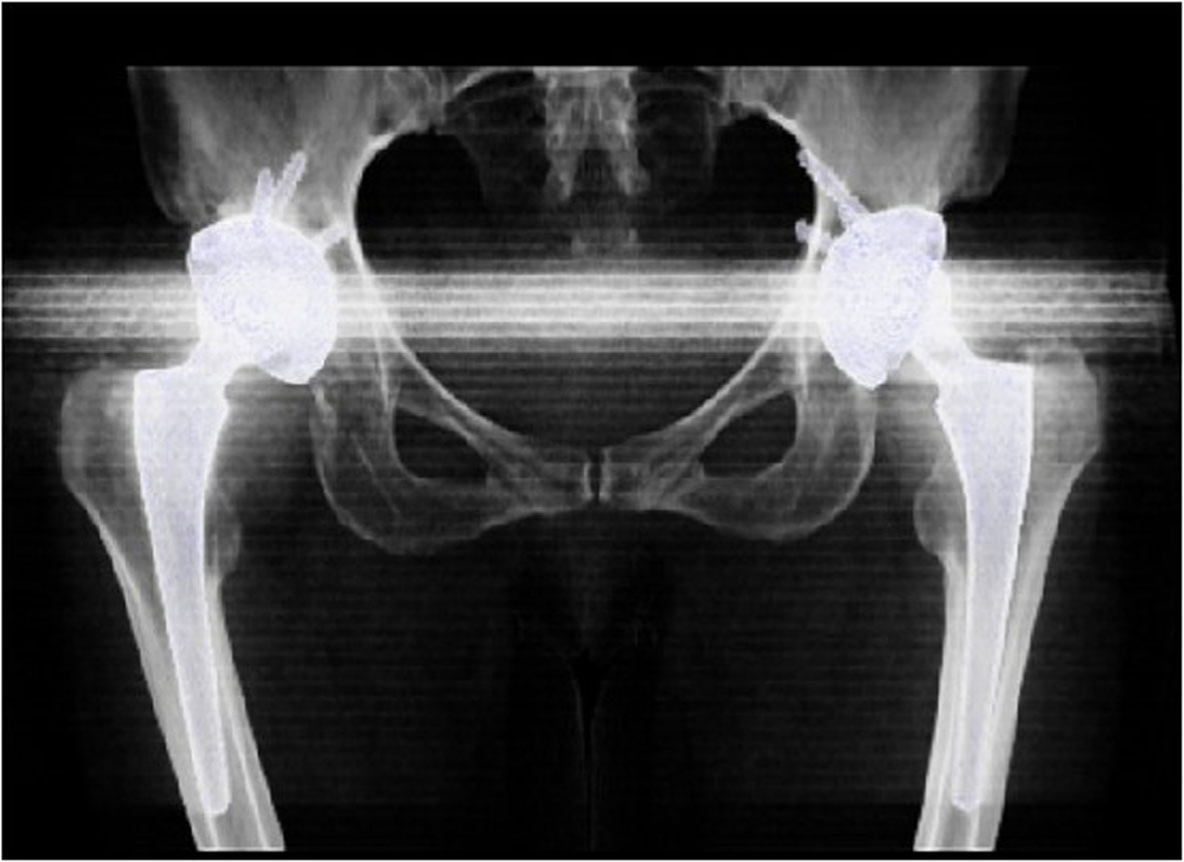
A PJI patient’s volume-rendered 3D CT imaging
